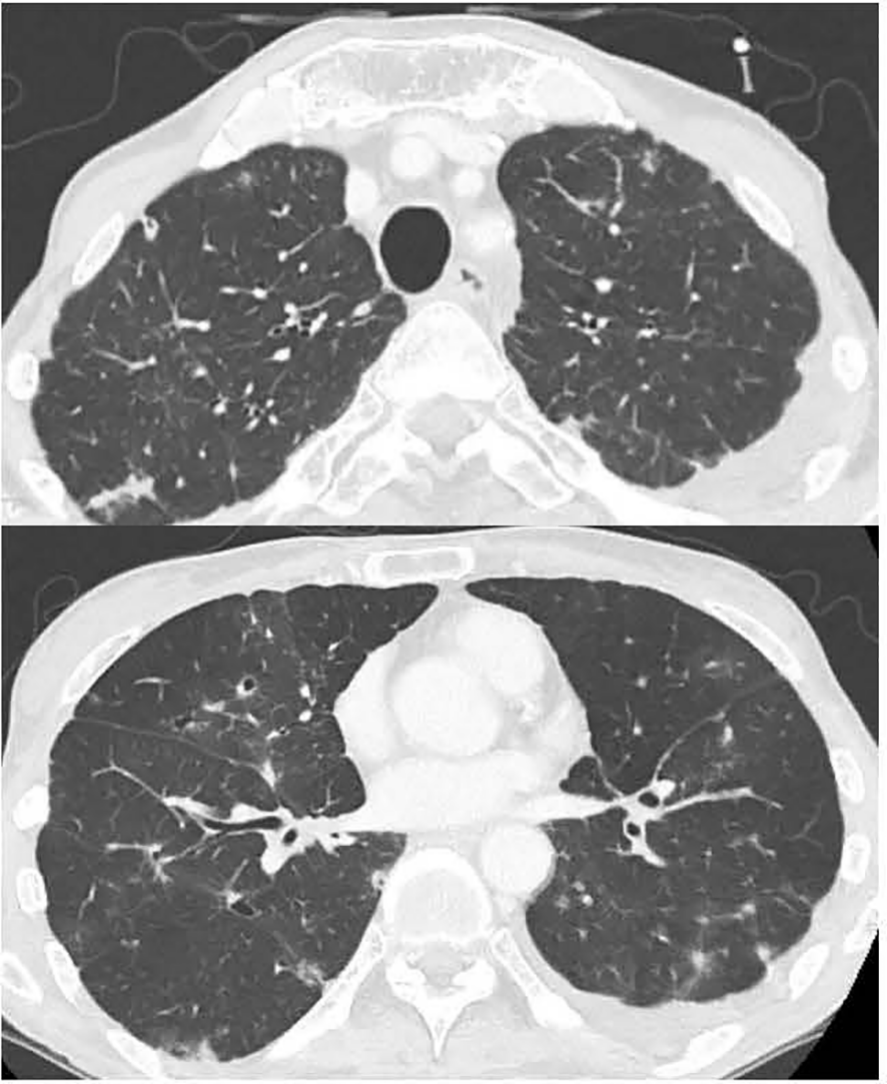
Chest CT showing the improvement in bilateral lung interstitial infiltrate shadows on day 21 of steroid treatment.
